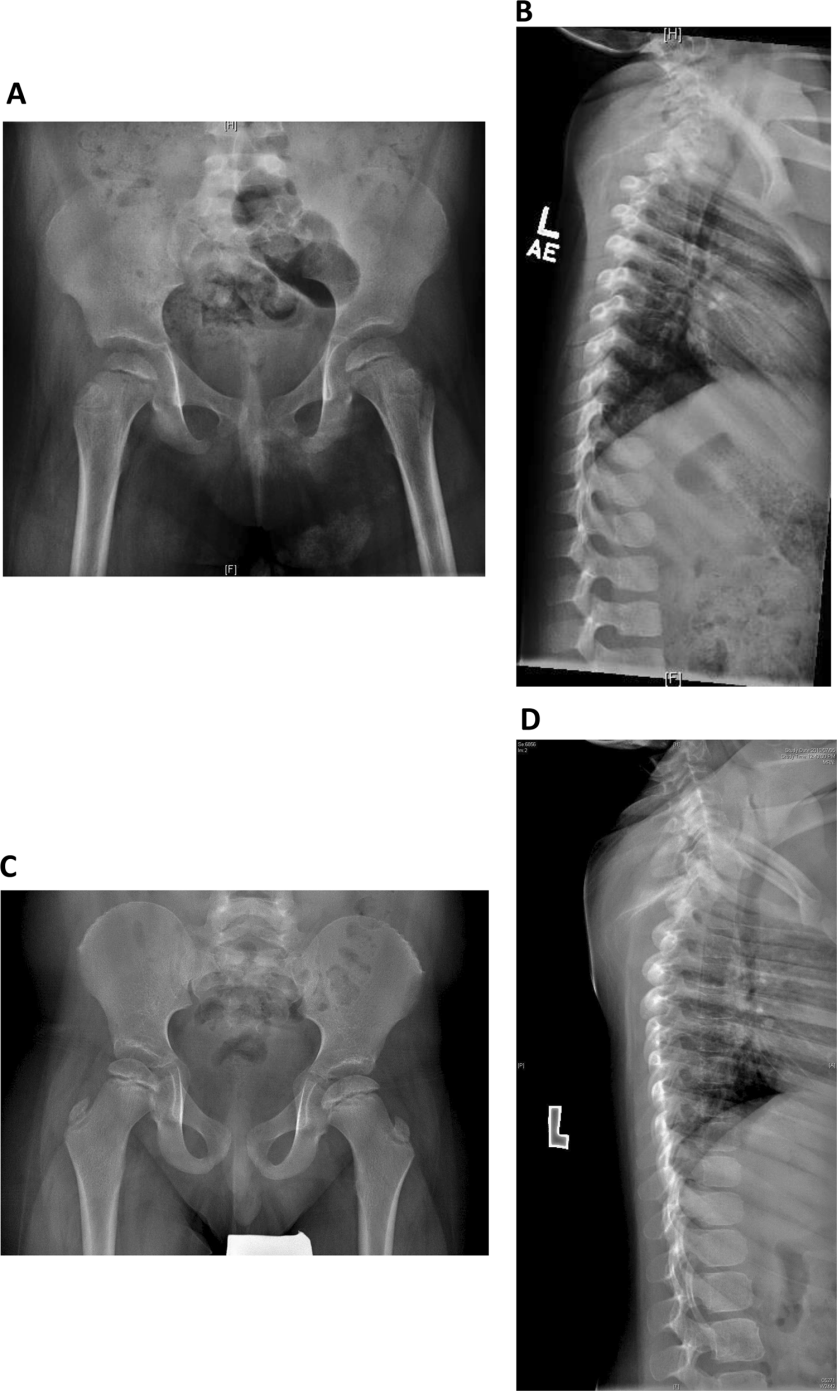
Skeletal features of Roifman syndrome in patients 1 and 2. a Pelvic X-ray of patient 1 featuring flattening of the humoral heads and shortening of the femoral necks, representing early stages of spondyloepiphyseal skeletal dysplasia. b Lateral spine X-ray of patient 1 featuring anterior vertebral notching of the lower thoracic vertebrae, and loss of lumbar lordosis. c Pelvic X-ray of patient 2 featuring bilateral small, flattened and slightly broadened femoral heads. d Lateral spine X-ray of patient 2 featuring loss of lumbar lordosis.
